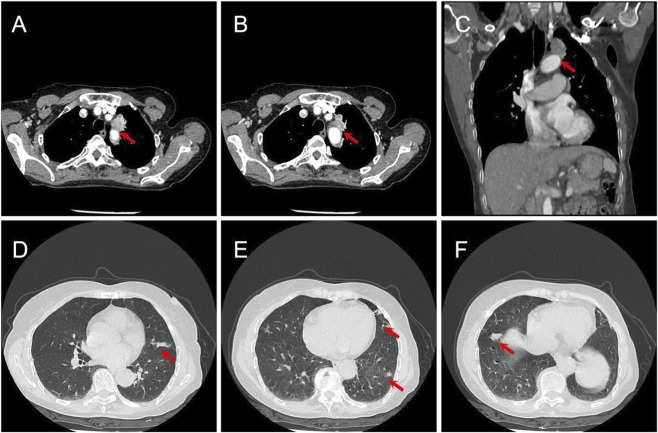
Baseline imaging findings at diagnosis. (A–C) Contrast-enhanced chest CT images show the primary left upper lobe tumor (arrows) with an indistinct border and loss of the fat plane adjacent to the mediastinum, consistent with clinical T4 (cT4) staging due to suspected mediastinal pleural invasion. (D–F) Multiple exudative lesions in both lungs, radiographically confirmed as COVID-19 pneumonia.
